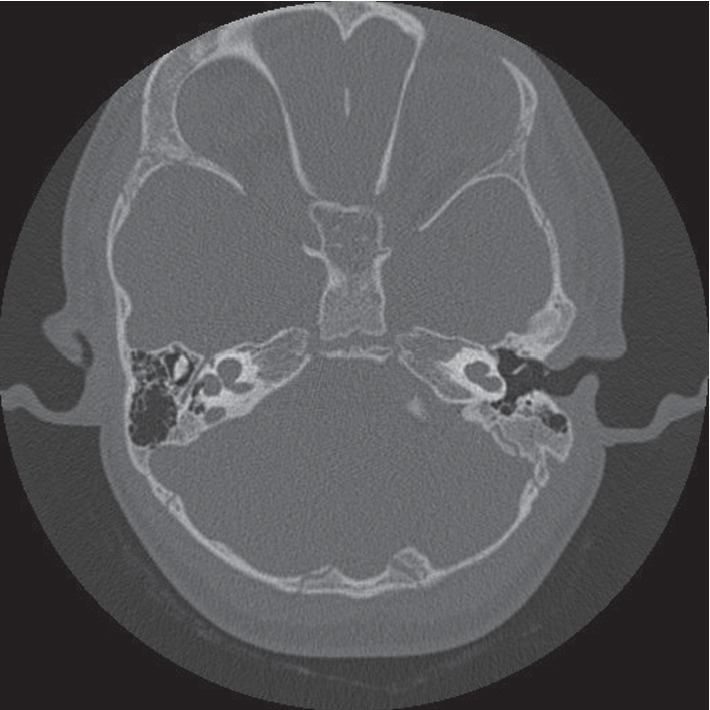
CT scan of the temporal bone with incomplete partition (IP-1).
